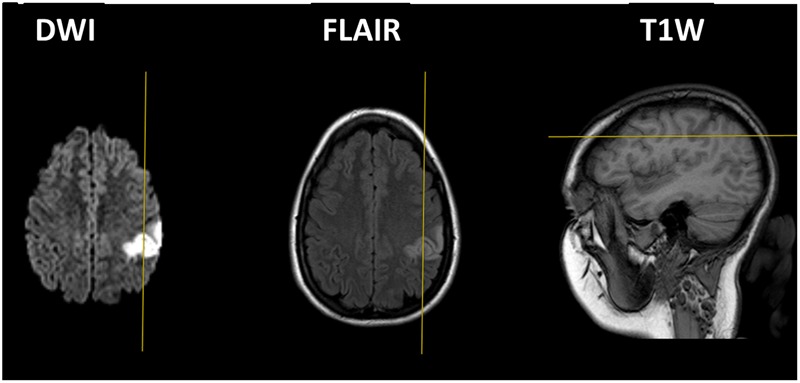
Magnetic resonance imaging (MRI) scans in DWI (left), flair (middle) and T1W (right), demonstrating an infarct in the left IPS. The right side of the picture refers to the left side of the brain. The left and middle pictures present horizontal sections and the right picture presents a sagittal section.
